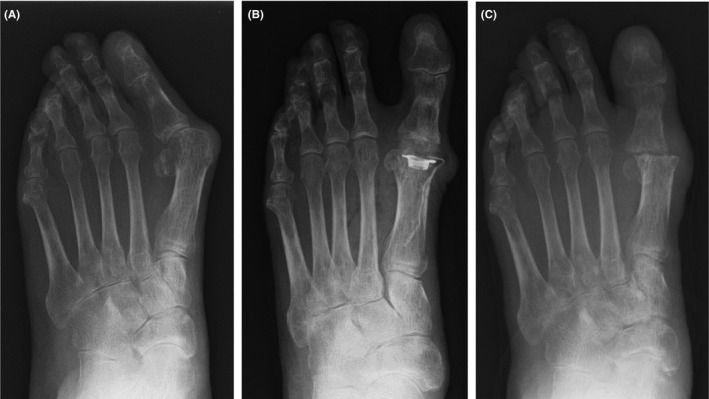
Anteroposterior foot radiographs. A, Patient had pronounced toe deformation. B, After Swanson arthroplasty, deformity of the hallux was corrected. C, At final examination, after removal implant, the hallux metatarsophalangeal joints demonstrated fibrous union
